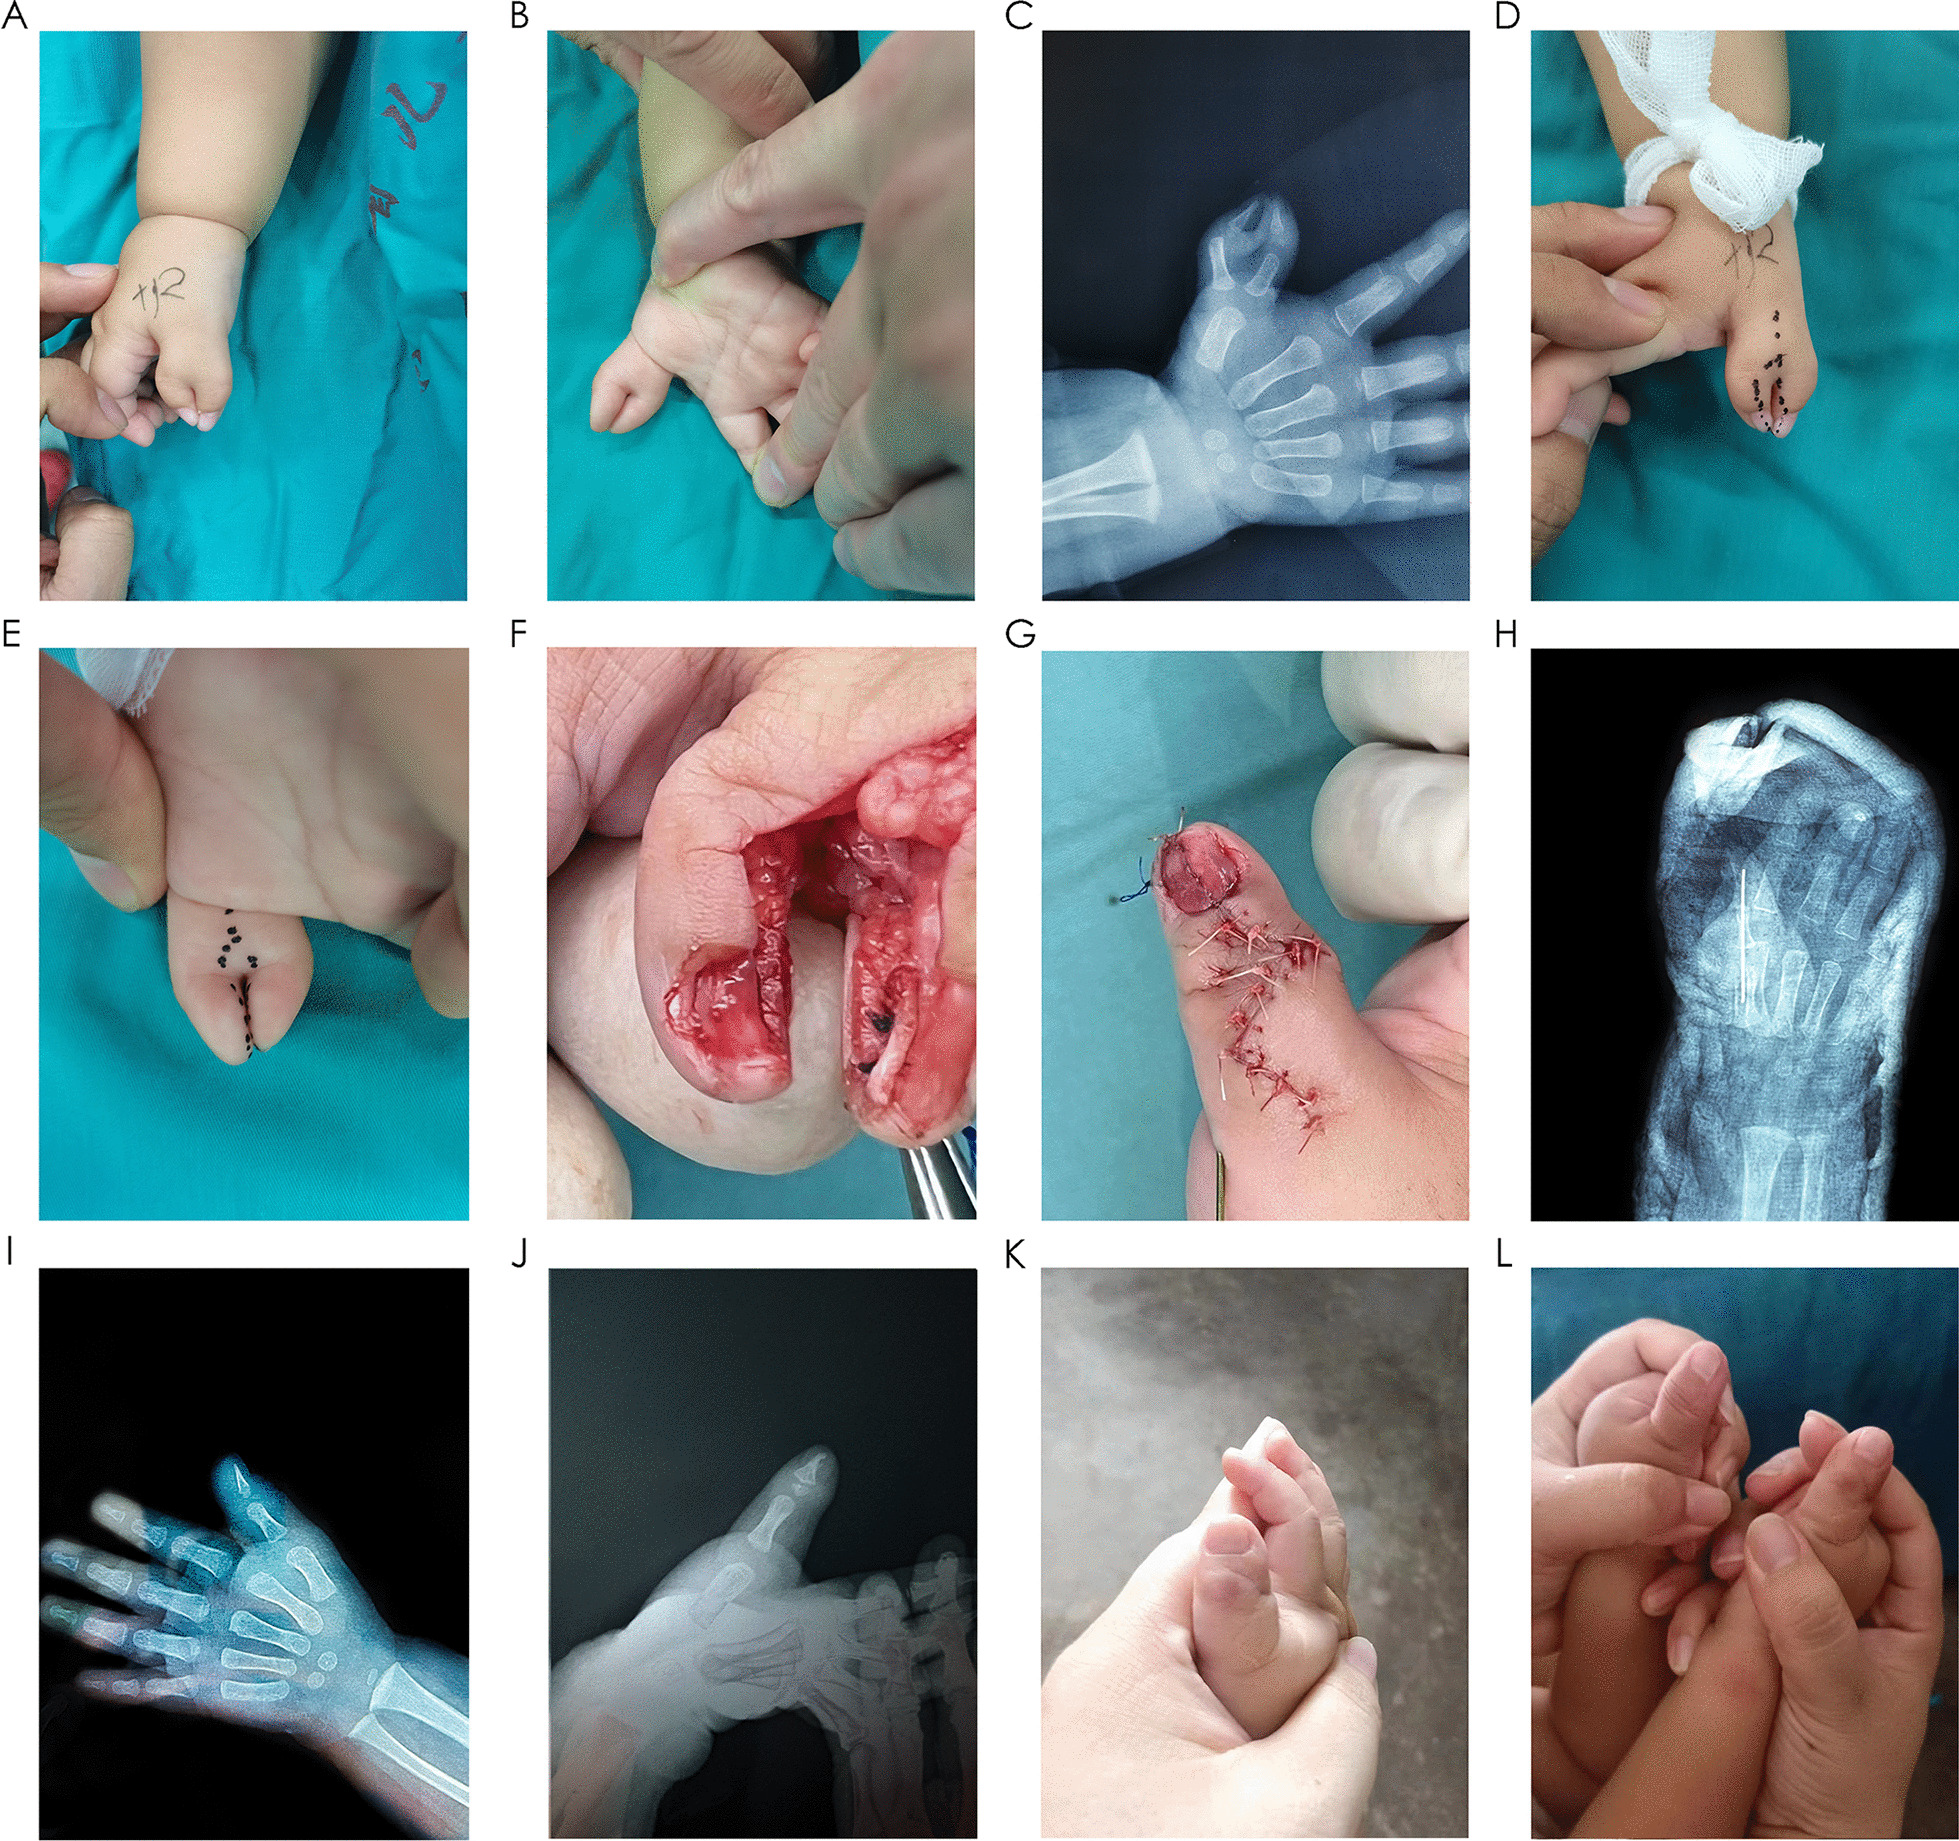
Case 5. A and B Preoperative of image. C Preoperative x-ray. D and E Draw incision. F Bone and nail assembly in surgery. G Immediate postoperative appearance. H Postoperative X-ray. I X-ray of 4 weeks after operation (K-wire removed). J Postoperative X-ray at 22 months. K and L Appearance following modified Bilhaut–Cloquet procedure at 22 months
